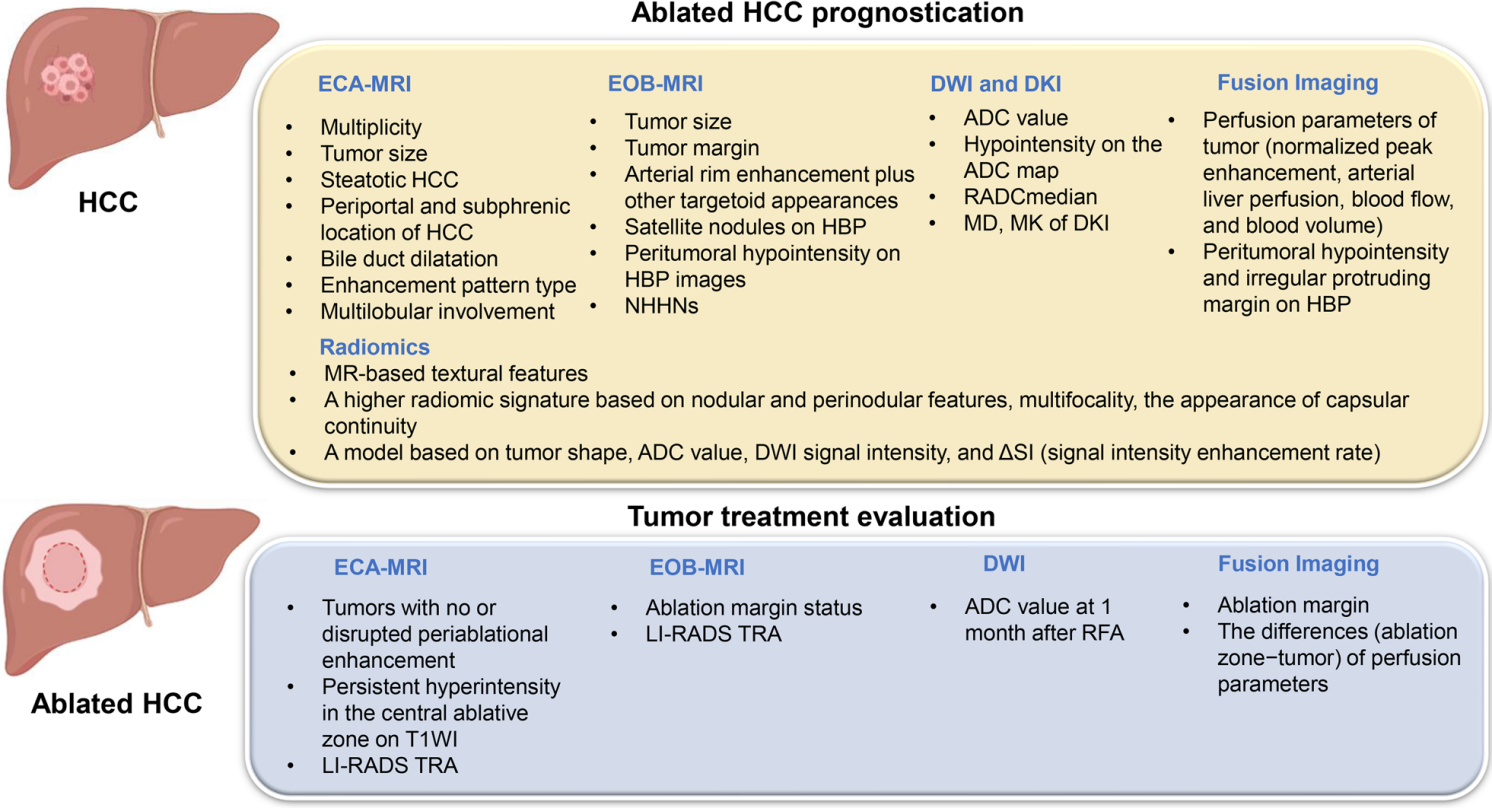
Published magnetic resonance imaging features for treatment response evaluation and prognostication of hepatocellular carcinoma after thermal ablation. RADC_median_ was defined as the ratio of ADC_median_ to the mean ADC of the non-lesion area
